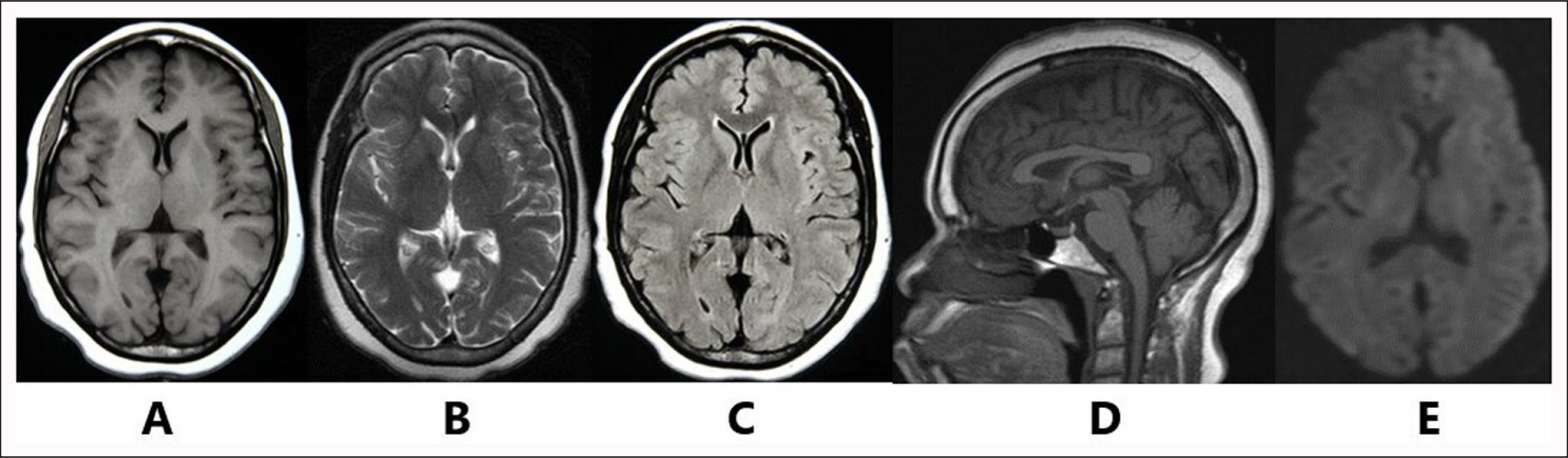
Brain MRI shows non-enhancing bilateral increased signal intensity on axial T1-weighted (A), axial T2-weighted (B) and axial T2-Fluid-attenuated inversion recovery (FLAIR)-weighted (C) images in periventricular regions, basal ganglia and internal/external capsules. Mild cerebral and cerebellar atrophy is exhibited in (A), (C), and (D). Sagittal T2-weighted image (D) shows normal corpus callosum, brainstem, and cervical-medullary junction. Axial diffusion-weighted image demonstrates no areas of restricted diffusion to suggest ischemia/infarction (E).
